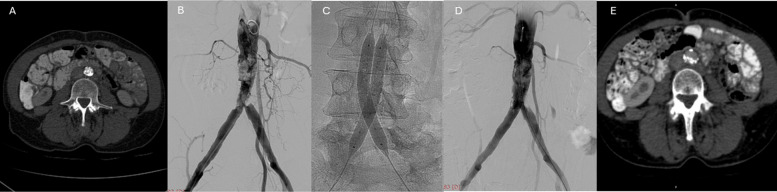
A Axial CTA demonstrating severe calcified aortic stenosis, B Baseline digital subtraction angiography (DSA) confirming concentric, heavily calcified narrowing of the aortic lumen, C Kissing intravascular lithotripsy (IVL) balloons positioned across the calcified aortic segment, D Final DSA showing restoration of the aortic lumen with preserved renal artery patency, E Axial follow-up CT demonstrating sustained luminal gain post-treatment
